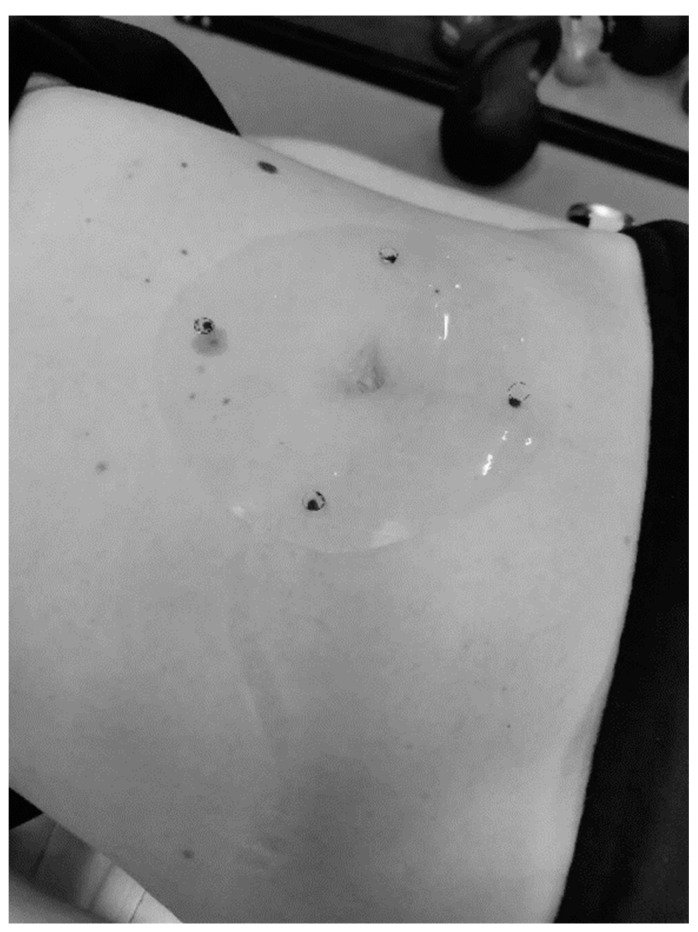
Template of ultrasound measurement points.
